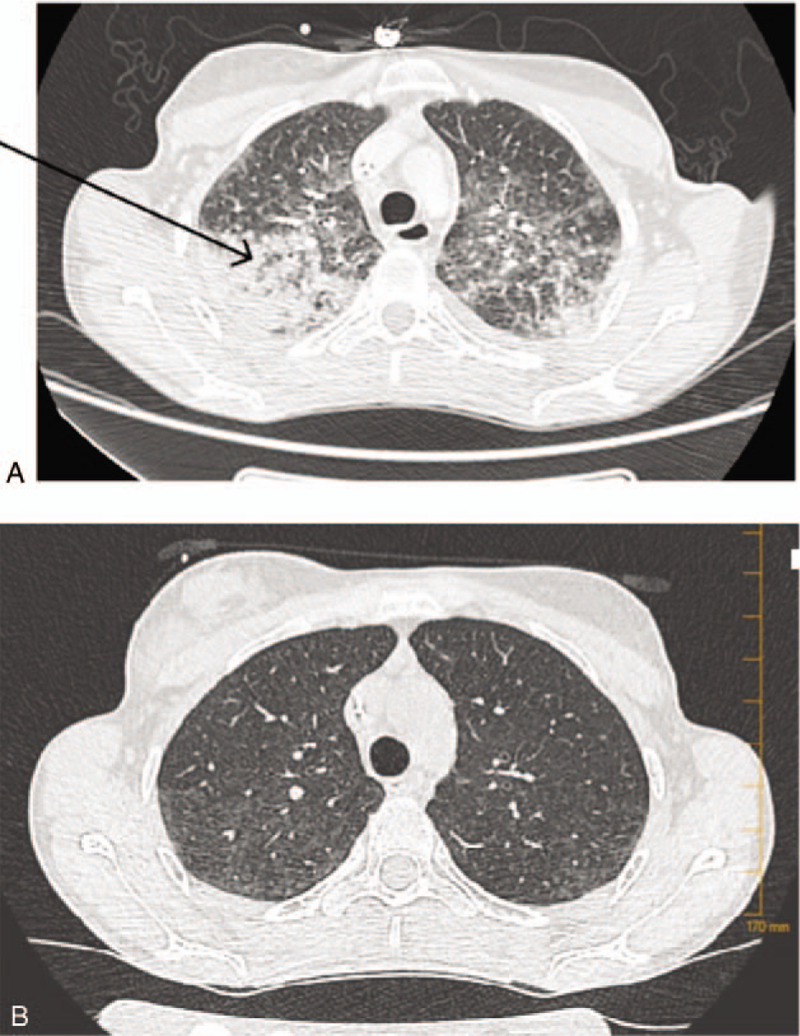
Pulmonary mucormycosis appearing as a mass with the halo sign on computerized tomography (CT) in 24-year-old woman after autologous hematopoietic stem cell transplantation for rapidly progressive diffuse cutaneous systemic sclerosis. A, CT scan on day 6, showing a bilateral parenchymatous condensation within the right upper lobe the reverse halo sign. B, CT scan on day 20 showing showed a nearly complete regression of bilateral parenchymatous condensation and disappearance of the reverse halo sign.
